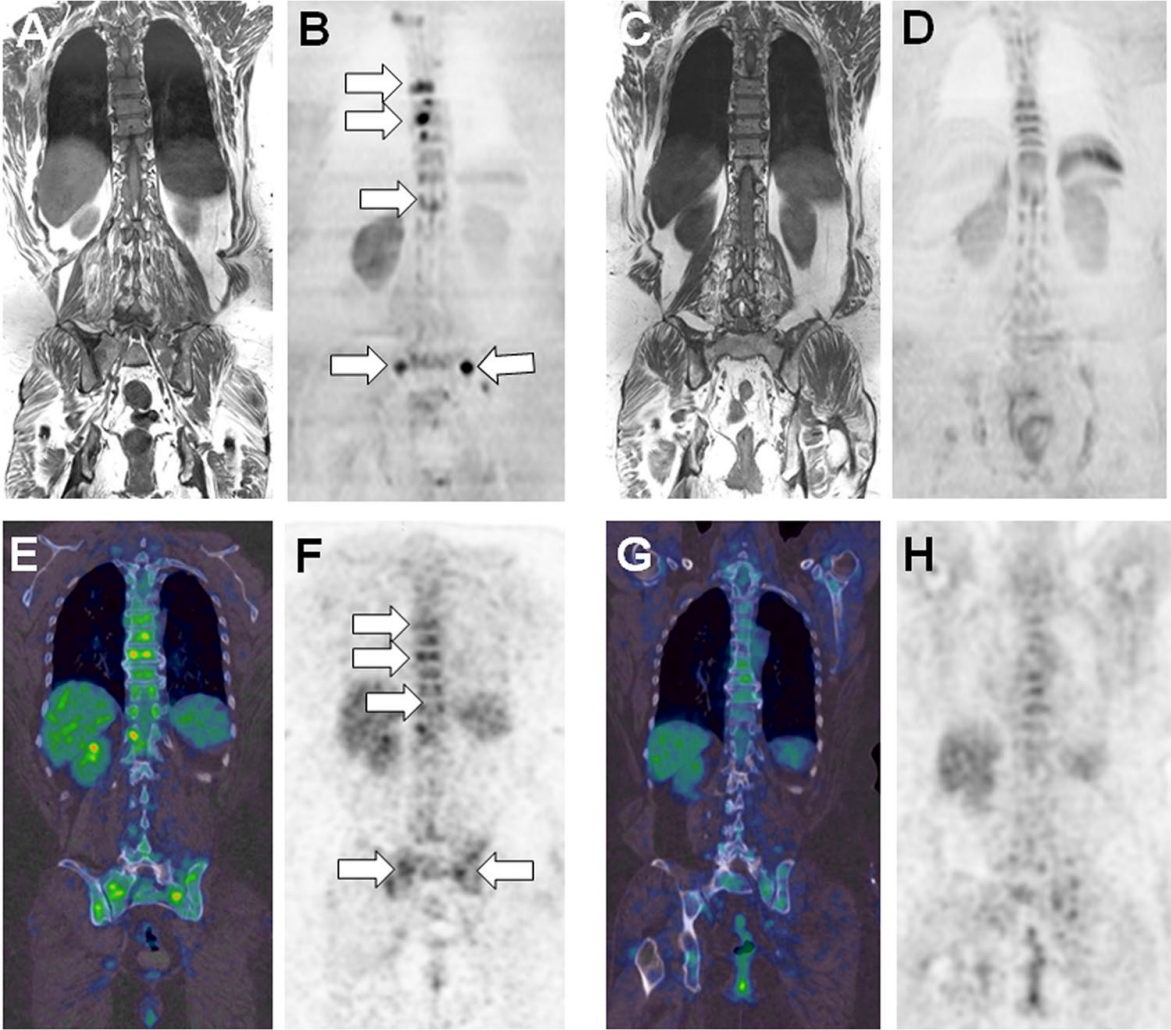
58 year-old woman with ND MM (IgA lambda, stage IIIA, ISS 2, with adverse cytogenetics (del 17p)). Comparison of WB-MRI and PET/CT findings at baseline and post-treatment (high dose cytotoxic treatment and ASCT). A–B Baseline MRI findings. Coronal T1 (A) and DWI (B = 1000 s/mm^2^) (B) MR images show active bone lesions within the spine and sacrum, barely seen on T1, much more evident on the DWI image (arrows in B). C–D Follow-up MRI. Coronal T1 (C) and DWI (B = 1000 s/mm^2^) (D) MR images show disappearance of all bone lesions. E–F Baseline PET/CT findings. Coronal fused PET/CT (E) and PET (F) show multiple foci with moderately increased [^18^F]FDG uptake (Deauville score 4) mainly involving the spine and sacrum (arrows in F) without evident lytic lesions or fractures on CT (not shown) (hottest bone lesion: T2 vertebral body, SUVmax 11.2; liver, SUVmax 4.2; mediastinal blood pool, SUVmax 2.8). G–H Following treatment, fused PET/CT (G) and PET (H) show disappearance of the focal PET uptake and reappearance of a homogeneous bone marrow uptake (Deauville score 3) suggesting a complete metabolic response (no residual disease at imaging) (T2 vertebral body, SUVmax 1.9; liver, SUVmax 4.0; mediastinal blood pool, SUVmax 2.7)
